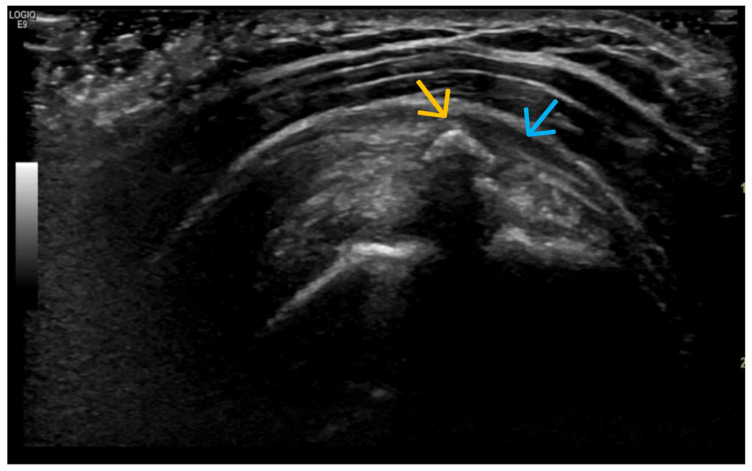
Ultrasonographic image of the shoulder (glenohumeral joint) with predominant visualization of the supraspinatus tendon in its longitudinal scan. The hyperechoic structure (orange arrow) in the middle of the tendon fibers, with the distally descending acoustic shadow, corresponds to a calcium-dense deposit. In addition to these findings, soft tissue changes are found—increased size and change in its echogenicity, i.e., tendinitis of the tendon, as well as discrete bursitis (blue arrow).
